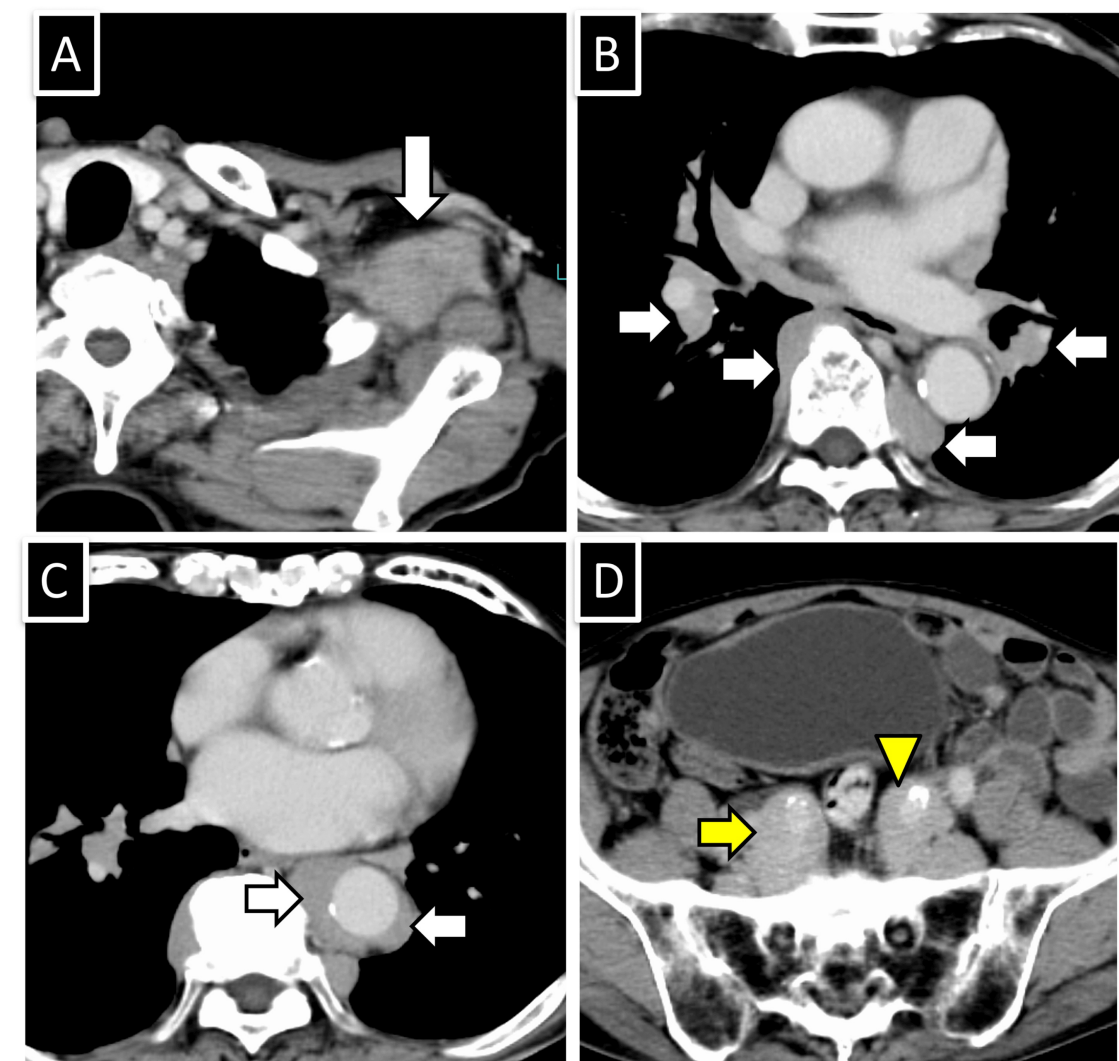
Contrast-enhanced CT before treatment. (A) Enlarged lymph node in the left axilla (arrow). (B) Soft tissue density masses observed in the hilum and paravertebral thoracic region (arrow). (C) Soft tissue density mass surrounding the descending aorta (arrows). (D) Soft tissue density masses around both internal iliac arteries (yellow arrow and yellow arrowhead). Atherosclerosis-induced calcification was observed in internal iliac arteries.
